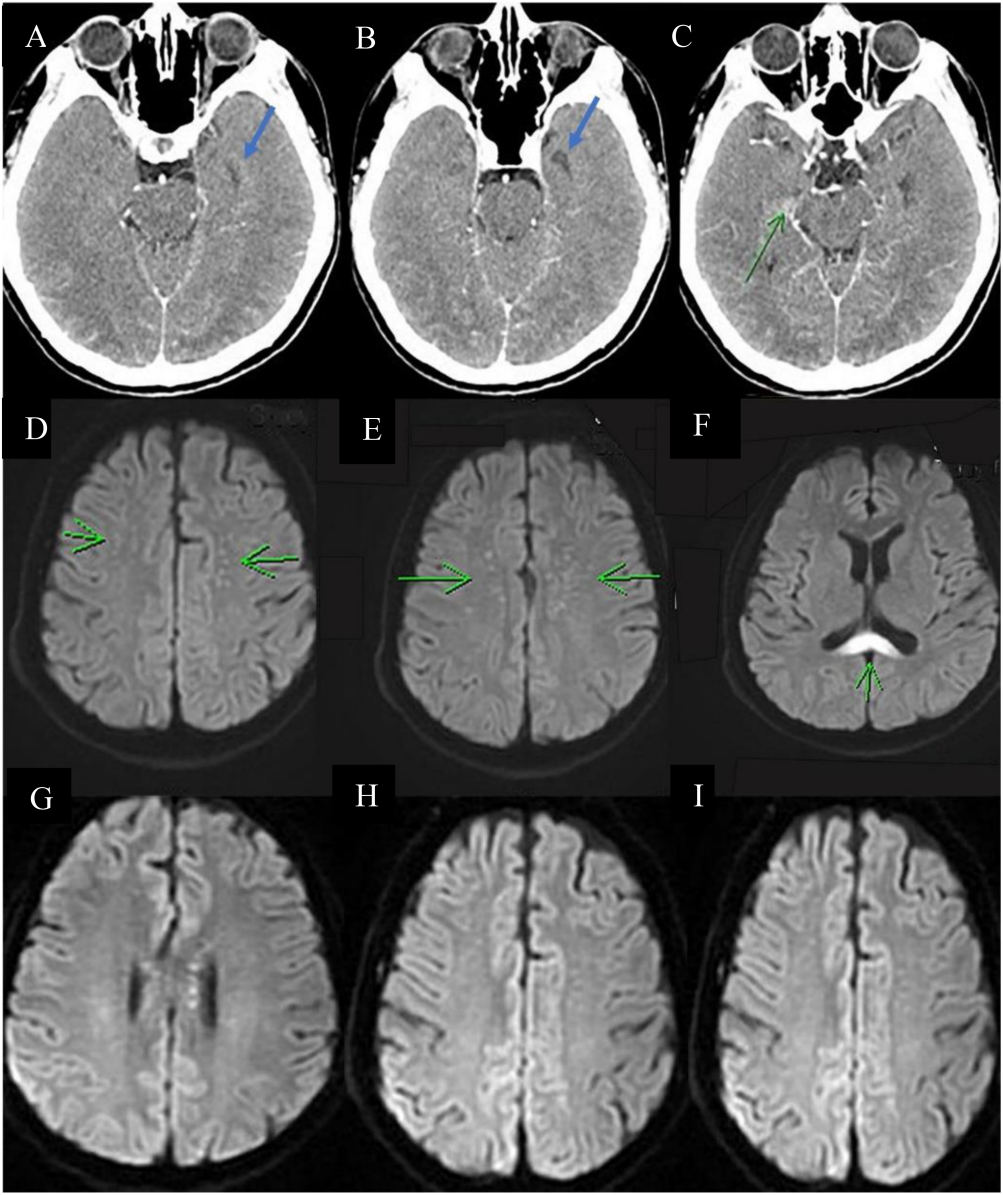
Serial images of the brain. Brain CT (A–C) showing a small hypodense lesion (blue arrow) and a small hyperdense lesion (green arrow) at the left superior frontal lobe and right temporal lobe, respectively. Brain MRI showing multiple tiny foci in bilateral centrum semiovale (green arrow in (D, E)) and diffuse restriction at the splenium of the corpus callosum [green arrow in (F)]. These findings are compatible with cytotoxic lesions of the corpus callosum, which indicate encephalitis. (G–I) Decreased diffusion restriction at the splenium of the corpus callosum and decreased abundance of multiple tiny foci in the bilateral centrum semiovale, suggesting regression of inflammation and CLOCCs.
